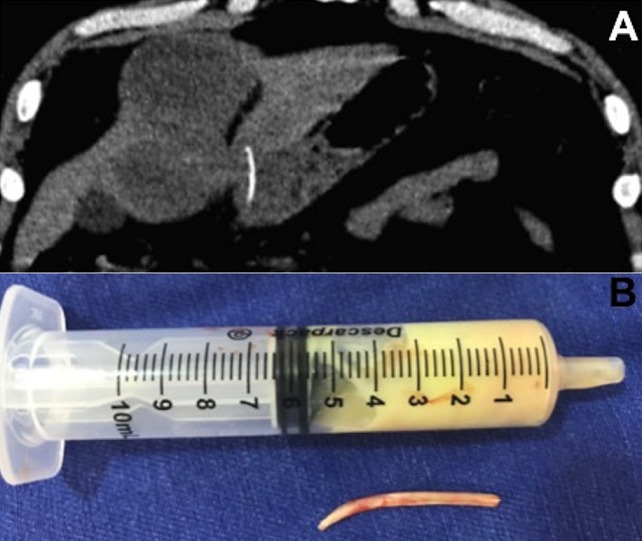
A) abdomen computed tomography showing a small linear structure with calcium density, transfixing the wall of the gastric antrum and penetrating the left hepatic lobe and an oval massive hypodensity; B) purulent fluid
